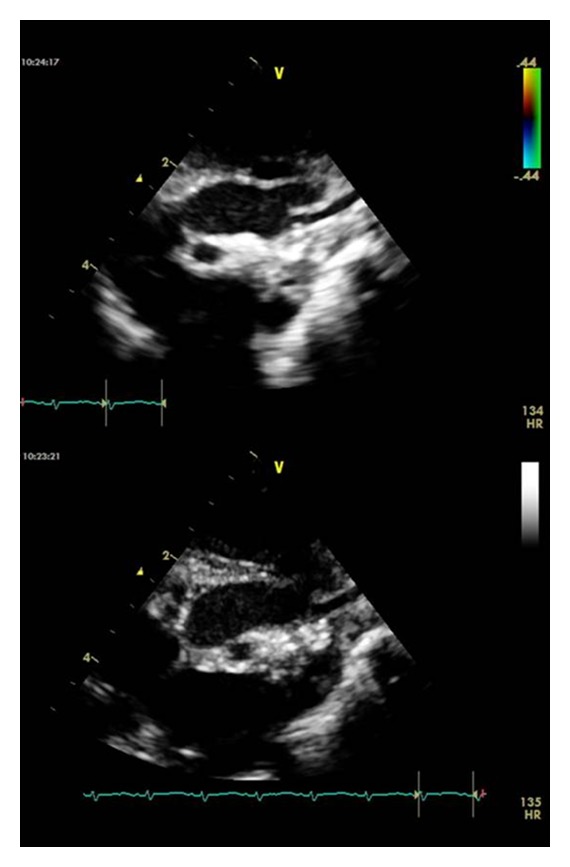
Echocardiogram showing the aortic arch with an echobright lesion within the lumen of the aorta just distal to the left subclavian artery.
